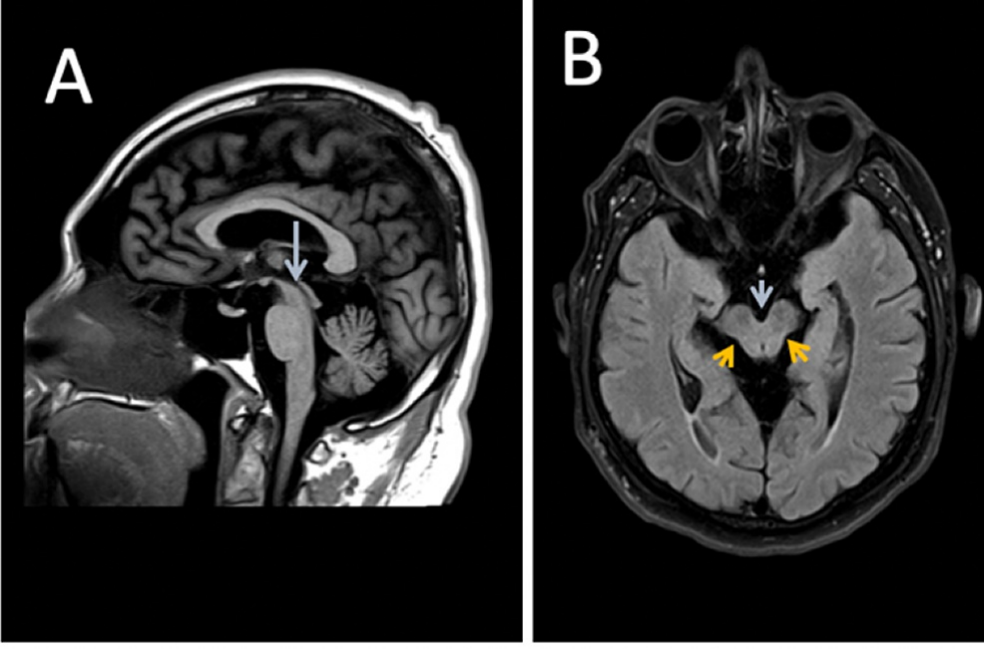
Neuroimaging findings in the patient Panel A: sagittal T1-weighted sequence shows prominent atrophy of the midbrain and superior colliculus, resulting in the concavity of the cranial mesencephalic margin. There is a "hummingbird sign" due to preserved pontine volume. Findings are most consistent with PSP. Panel B: axial fluid-attenuated inversion recovery (FLAIR) sequence MRI also demonstrates a concave dorsolateral midbrain margin. The corresponding axial images reveal a concave dorsolateral midbrain margin (orange arrows) and widening of the interpeduncular cistern (blue arrow) MRI: magnetic resonance imaging; PSP: progressive supranuclear palsy
